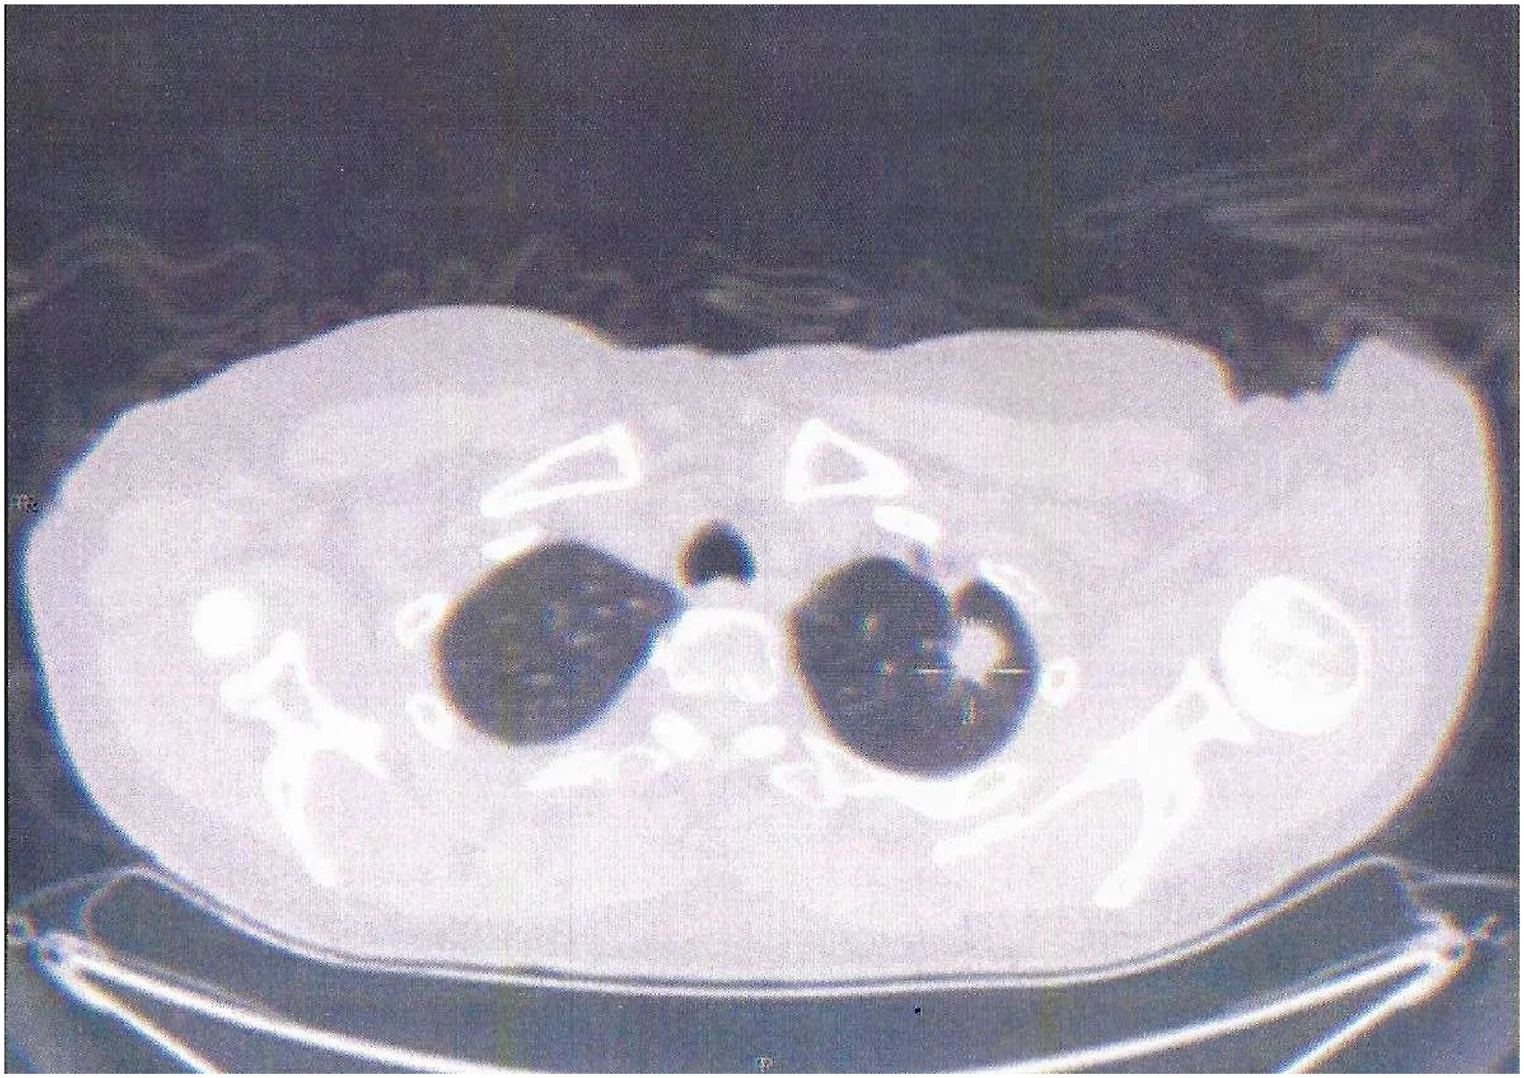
PET-CT with 18F-FDG (December 20, 2025).
